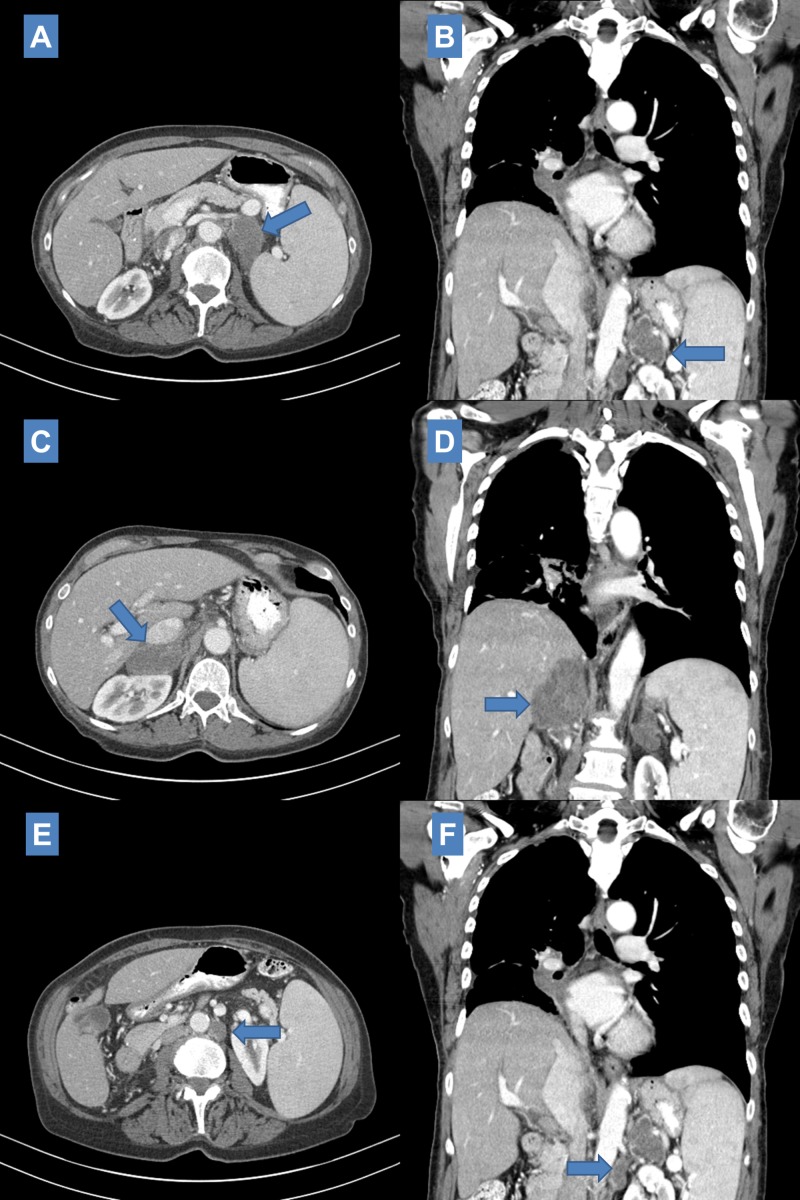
One month post SBRT CT scan showing decrease in size of left adrenal metastasis (4.4 cm x 2.3 cm SBRT: Stereotactic body radiation therapy; CT: Computed tomography.
